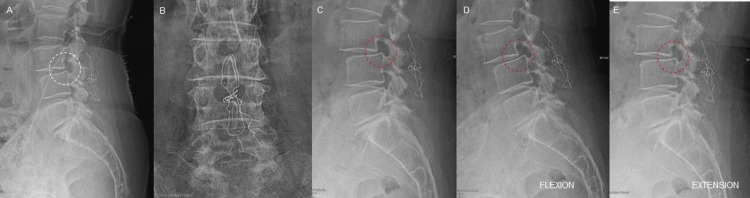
Case 1 Progression of Slippage X-Rays Case 1: Post-ILP radiographs lateral (A) and AP (B) views taken two months after the first surgery showed preserved segmental motion and minimal translation at the L3-L4 level (A, white circle). Six months postoperative lateral radiographs in neutral (C), flexion (D), and extension (E) position showing slippage progression and dynamic angular instability secondary to the spondylolisthesis at the previously mentioned level (encompassed in the red circle). ILP: interspinous ligamentoplasty, AP: anteroposterior.
